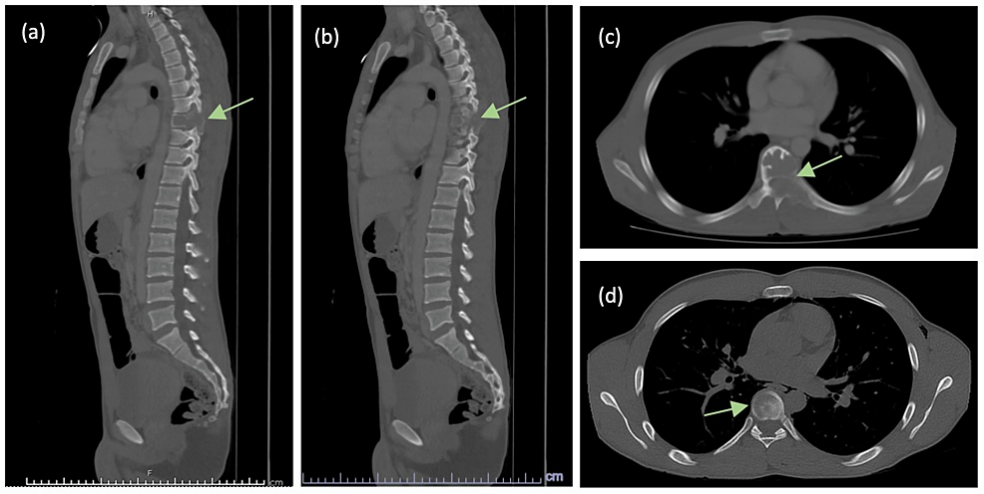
Computed tomography images demonstrating an expansile soft tissue mass in the eighth thoracic vertebra. (a) Sagittal view of soft tissue mass involvement of the T8 vertebral body, left pedicle, and spinous process. (b) Sagittal view demonstrating soft tissue mass extension into surrounding structures. (c) Axial view demonstrating involvement in the T8 left pedicle, left transverse process, and left lamina. (d) Axial view highlighting involvement of the T8 vertebral body.
